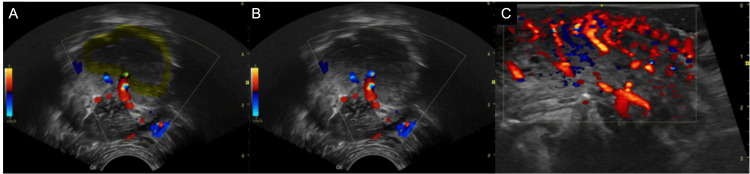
Color Doppler ultrasound of the dorsal tongue. (A,B) Multiple prominent, tortuous vascular channels within the tongue musculature, showing both arterial and venous flow with arteriovenous shunts. (C) Longitudinal view demonstrating extensive vascularity involving the mid and anterior thirds of the tongue.
